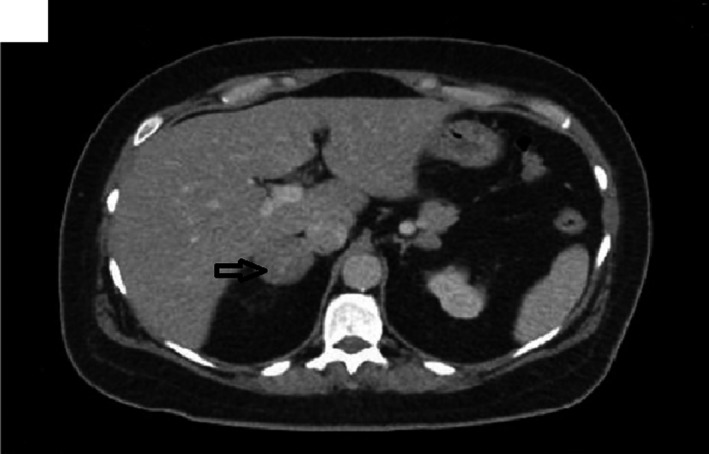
CT of the abdomen with contrast showing 4 cm right adrenal adenoma
